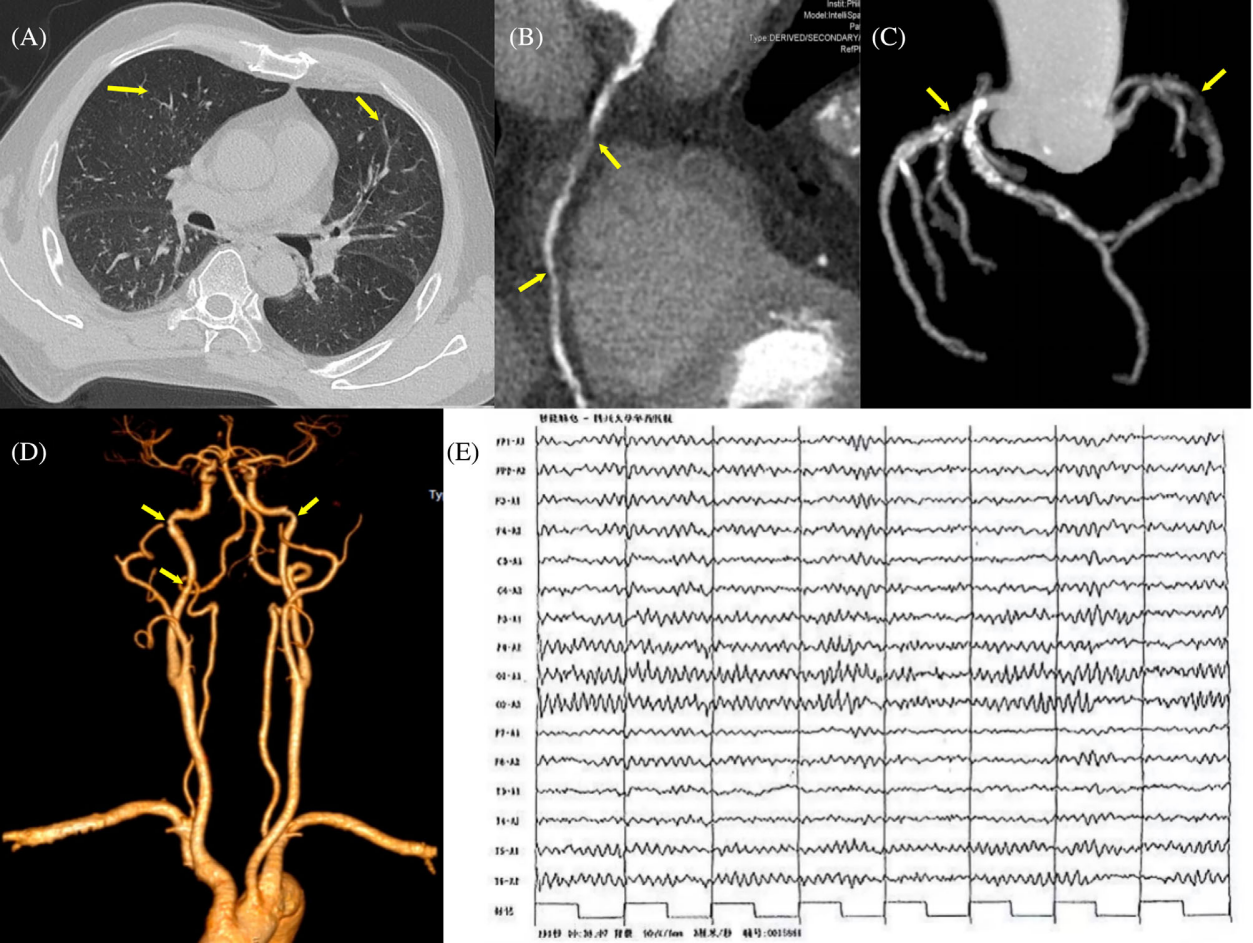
(A) Chest CT showing a “barrel chest” and emphysema (the yellow arrows). (B), (C) Coronary CT angiography showing significant calcification in the left coronary artery, including the anterior descending artery and the circumflex branch (B), as well as 80% stenosis in the right coronary artery (C) (the yellow arrows). (D) Neck CT angiography showing mild stenosis of the bilateral internal carotid arteries (the yellow arrows). (E) Electroencephalogram taken during a patient‐reported cough syncope episode showing normal results. CT, computer tomography.
